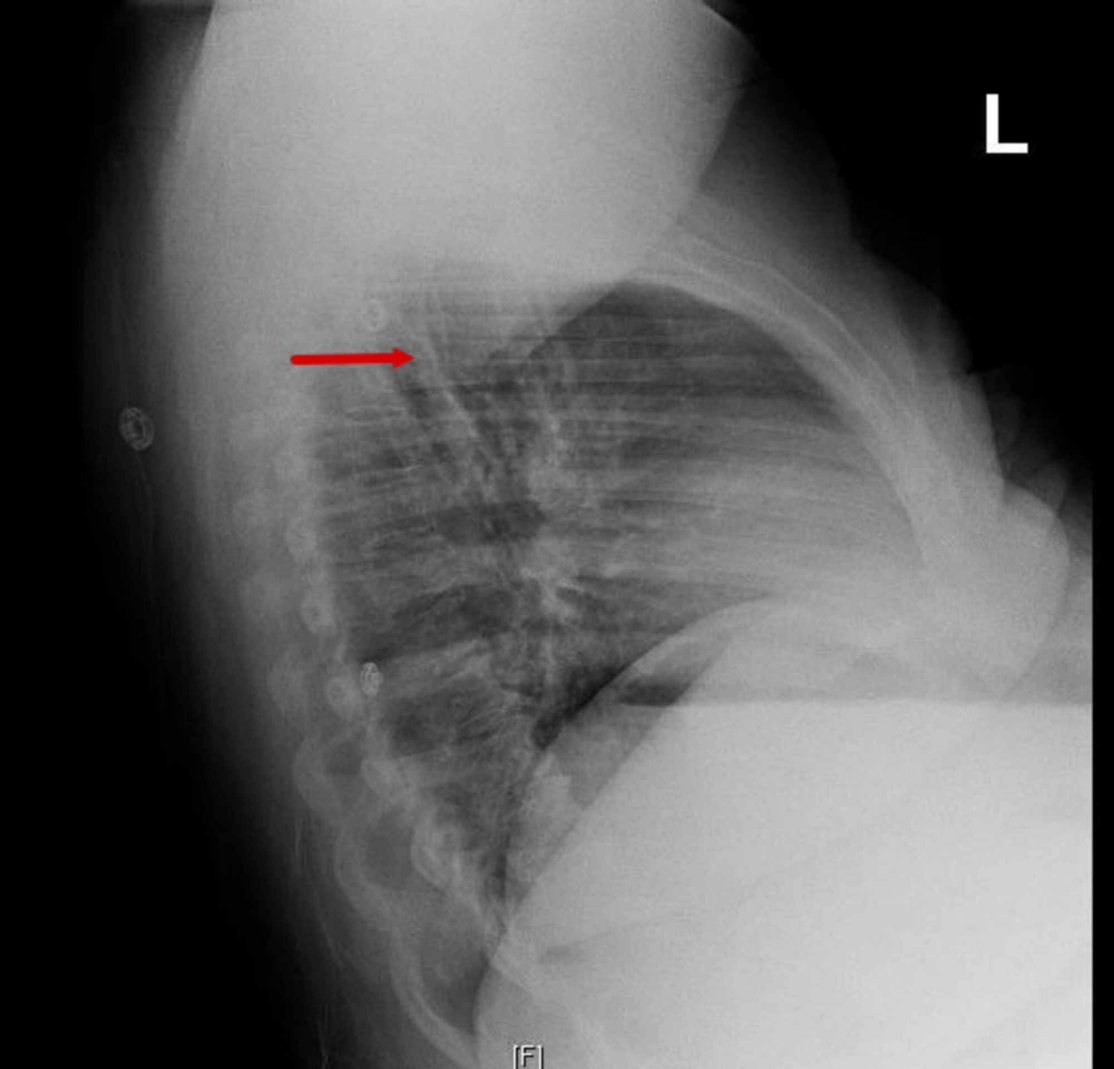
Lateral view of chest X-ray with findings suspicious for right upper lobe pneumonitis.
